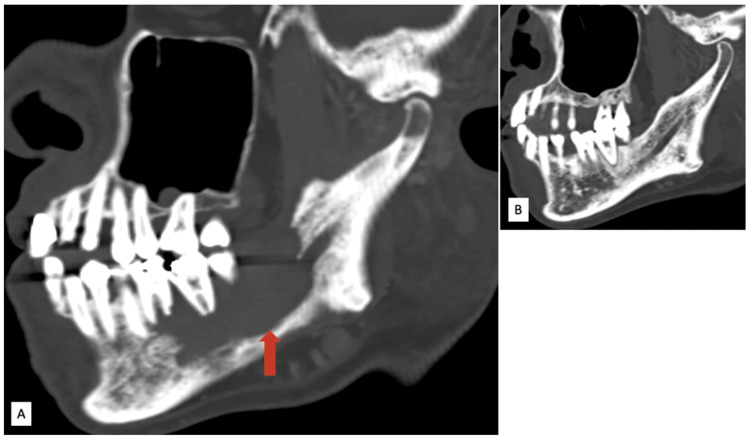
Contrast-enhanced CT neck images in the agittal oblique plane. (A) Lytic lesion in the right hemimandible exposing the right mandibular canal. (B) Normal left hemimandible with an intact mandibular canal for comparison.
